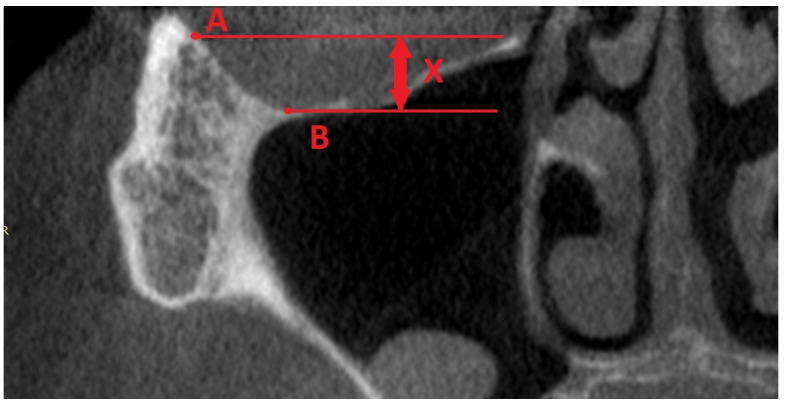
Zygomatic Orbital Floor (ZOF) classification. The most lateral point (A) was in the lower wall of the orbit. Reference points in the frontal plane on CBCT were the lowest (B). Then, two simple parallel lines perpendicular to the basal line of the transversal plane were prolonged, and the distance between them (x). Thanks to ZOF classification the risk of orbital damage is significantly lower.
